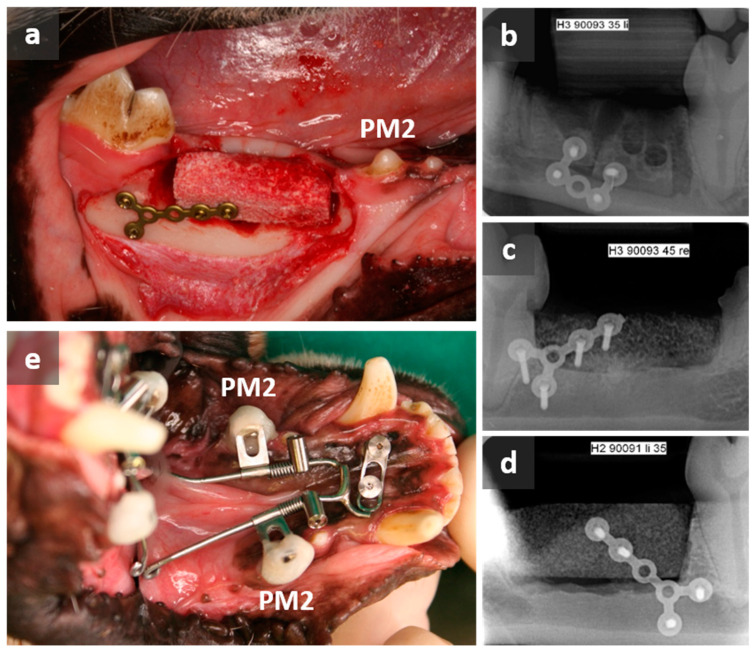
Intraoral images and X-rays of postoperative situations. Fixed BRMs positioned in the distal direction of the second premolar (PM2) (a). X-rays directly after surgical procedure of the autograft (b), xenograft (c), and hydroxyapatite (d). Bilateral distalization of PM2 started seven weeks after insertion of BRMs with an orthodontic appliance, which corresponded to the Beneslider system (e).
